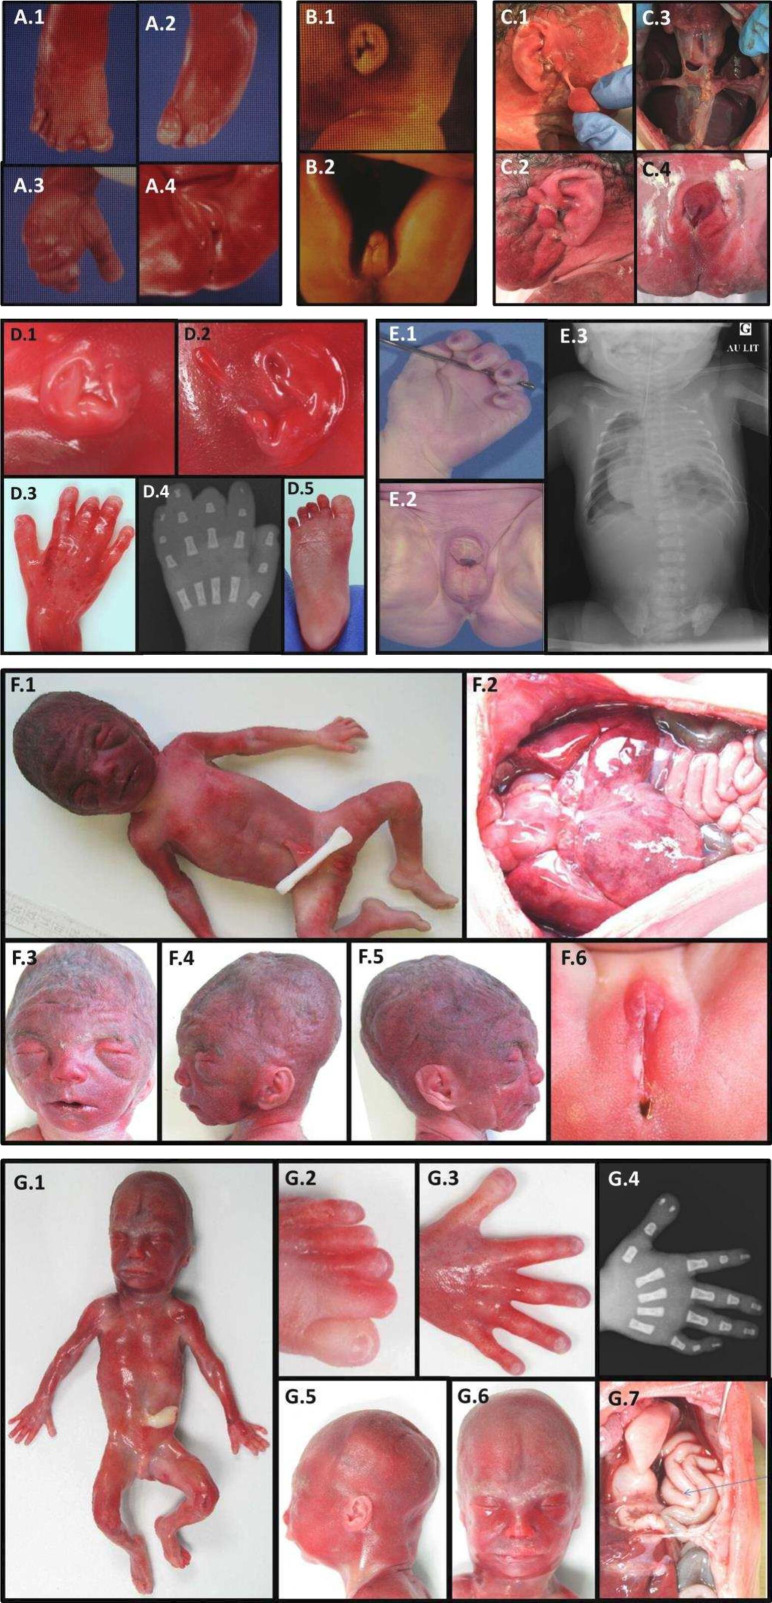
Postmortem findings. Patient 1 (A.1–A.4) at 23+5 GW, showing pre-axial polydactyly of the right foot (A.1), overlapping toes of the left foot (A.2), large thumb (A.3) and DSD (A.4). Patient 2 (B.1–B.2) at 37 GW showing small low-set ears (B1) and DSD (B.2). Patient 6 (C.1–C.4) at 31 GW. Note the large bilateral pre-auricular skin tags (C.1, C.2), bilateral posterior CDH with intrathoracic left and right sides of the liver (C.3), DSD with hypospadias and empty scrotum (C.4). Patient 7 (D.1–D.5) at 20 GW. Note a small right ear (D.1), two left pre-auricular skin tags (D.2), broad thumbs, short distal phalanges of the second and third fingers (D.3, D.4), broad halluces and short distal phalanx of the third toes (D.5). Patient 8 (E.1–E.3) at 38 GW, showing single transverse palmar crease (E.1), DSD with hypospadias (E.2), left CDH with ipsilateral lung hypoplasia and intrathoracic digestive tract on X-rays (E.3). Patient 9 (F.1–F.6) at 25 GW. Note abnormal facial shape with hypertelorism, anteverted nares, low-set ears and retrognathism (F.1, F.3–F.5), CDH (F.2) and DSD (F.6). Patient 10 (G.1–G.7) at 21 GW, showing DSD (G.1), microretrognathism (G.5–G.6), broad hallux and two to three-toe syndactyly (G.2), duplicate right thumb (G.3, G.4) and left CDH with intrathoracic digestive loops (G.7).
